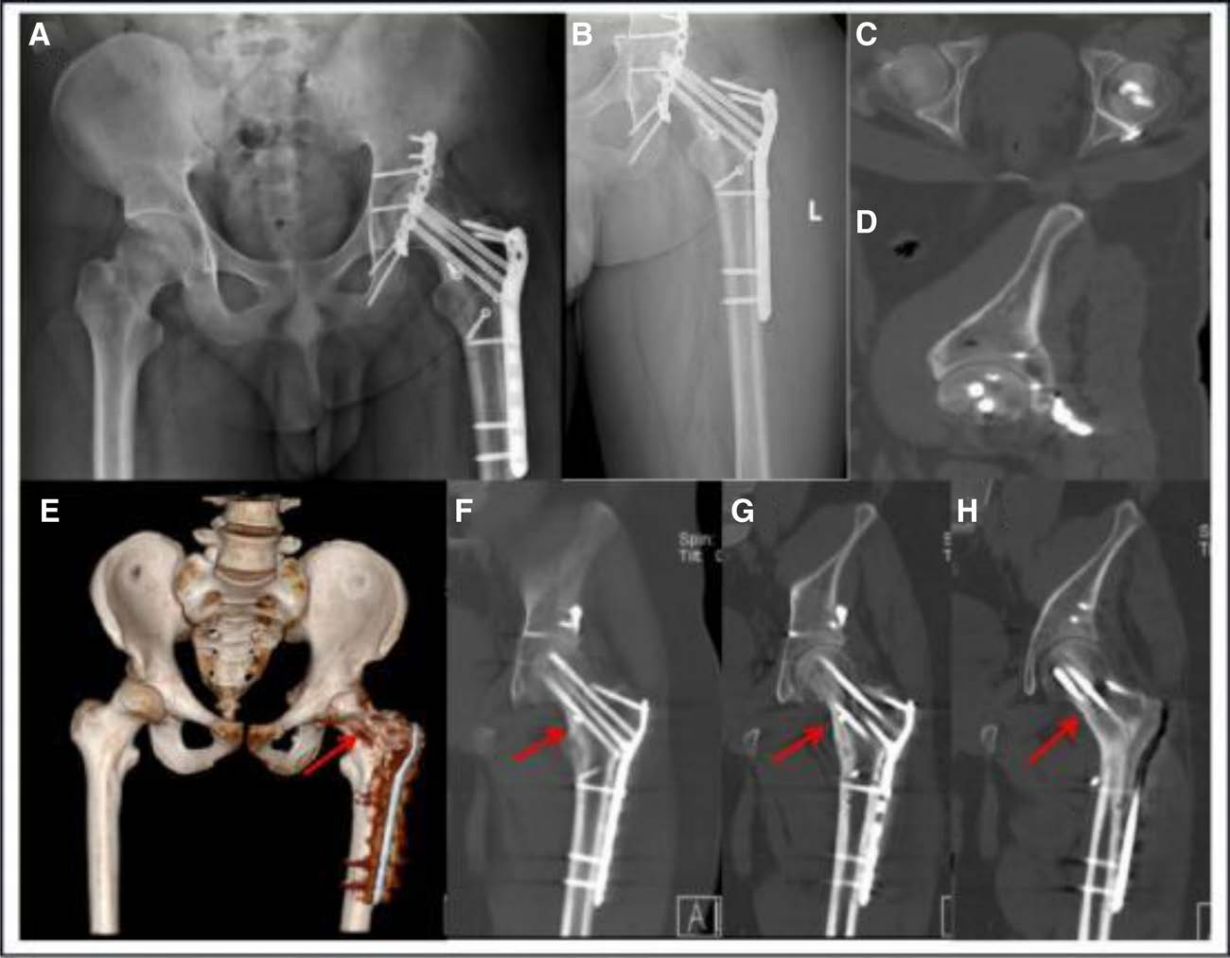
The patient review 6 months after surgery showed that the internal fixation position was consistent with that after surgery, with no collapse and necrosis of the femoral head, no sclerosis of the acetabulum, large callus formation, and a more blurred fracture line than before. (A) Anteroposteric DR image of the pelvis; (B) anteroposterior DR image of the left hip joint; (C) CT scan image of hip joint cross-section; (D) sagittal CT scan of the hip joint; (E) anteroposteric CT 3-dimensional reconstruction of the pelvis, the length of the femoral neck was basically the same on both sides, and there was more callus formation at the proximal end of the left femur; (F, G, H) Sagittal CT scan of the left hip joint, the fracture line of the proximal femur was blurred, the fracture of the femoral neck was well healed, and no collapse or necrosis of the femoral head was observed. DR = digital radiography.
